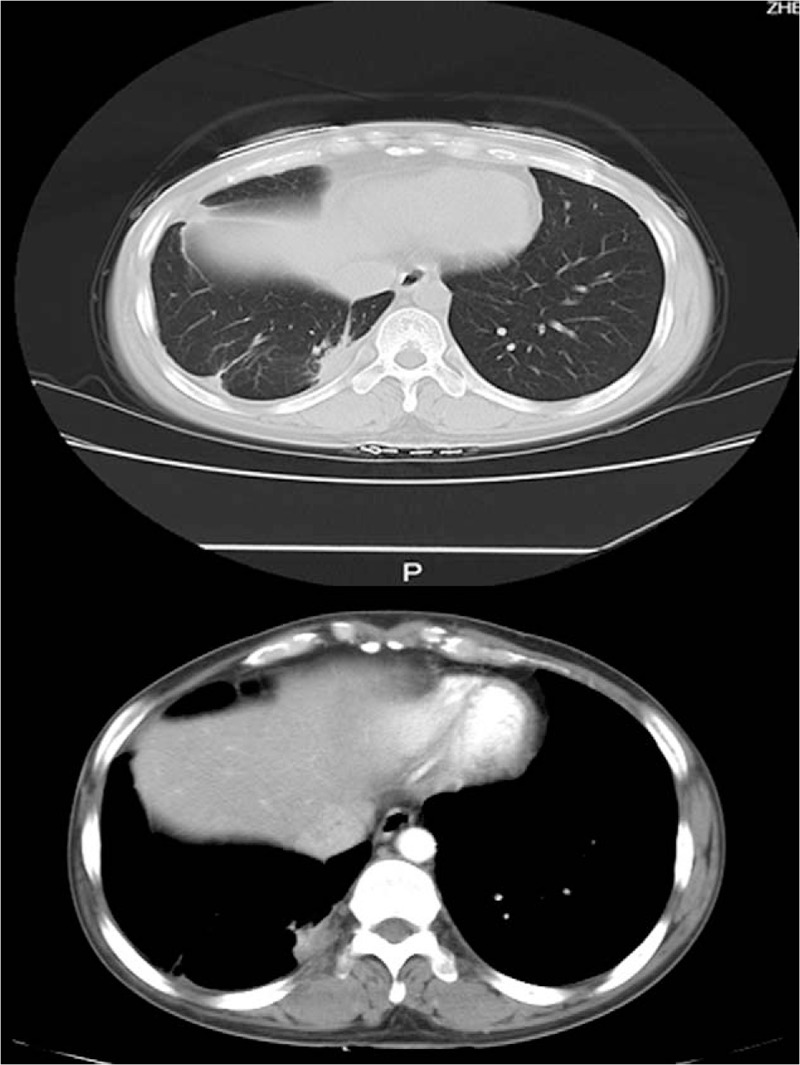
Computed tomography showed that mass in the right pleura.
